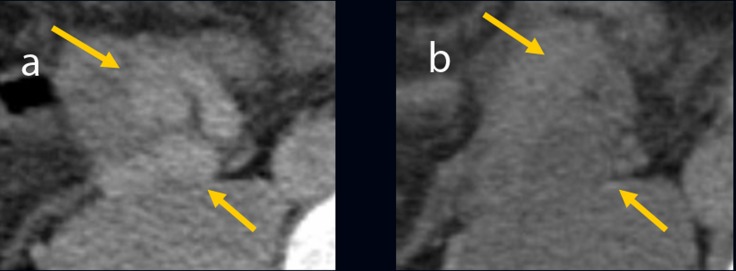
(a) Computed tomography image of ileal vessels before balloon occluded retrograde transvenous obliteration. (b) The ileal vessels are no longer visible following balloon occluded retrograde transvenous obliteration.
